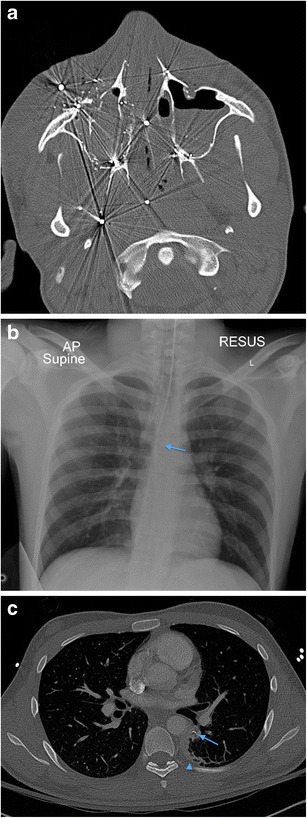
a Axial non-contrast CT face image in a 19-year-old male victim of a shotgun injury to the orbito-facial region. Multiple shotgun pellets are identified in the malar soft tissues, nasal fossae, maxillary sinuses, nasopharynx and masticator space, particularly on the right at this axial level. Fracture fragmentation of the right maxillary sinus, malar and retroantral haematoma and antral haemorrhagic fluid are present. The involvement of the nasal, nasopharyngeal and oral (the latter not shown) airway raises potential for distal migration of pellets (as well as of in-driven tissue fragments) through the upper aerodigestive tract into the lower respiratory tract and gastrointestinal tract. b Plain chest radiograph in the same patient as in 13a performed during trauma survey on admission demonstrates distal migration of a shotgun pellet (*blue arrow*) into the mid-oesophagus (confirmed on CT chest assessment [not shown]). The patient is intubated. c Axial image of a contrast-enhanced CT thorax of the same patient as in a and b, performed 12 h later also confirms aspiration of a shotgun pellet (*blue arrow*) associated with distal subsegmental left lower lobe atelectasis (*blue arrowhead*). There had also been further distal intra-oesophageal migration of the mid-oesophageal shotgun pellet (image not shown)
